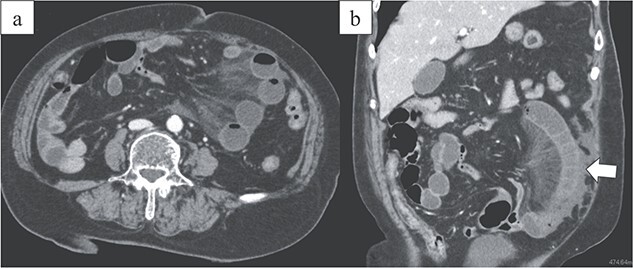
Images from enhanced CT. (a) Arterial-phase axial CT shows a hypo-enhancing small bowel in the left lower abdominal quadrant. (b) Arterial-phase coronal CT coronal enhanced CT shows a closed-loop obstruction of the hypo-enhancing small bowel (arrow).
